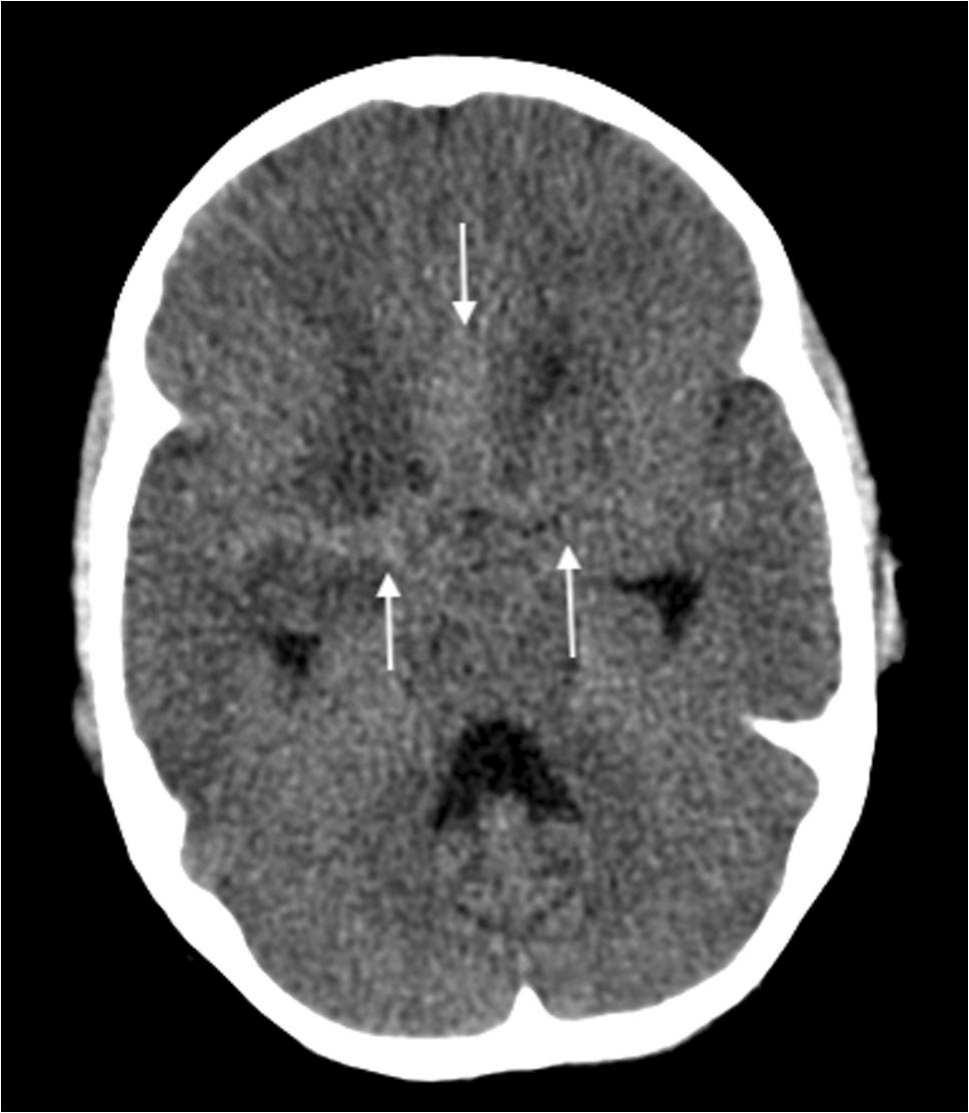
Non-contrast axial computed tomography of the brain in a 30-month-old boy with tuberculosis demonstrates hyperdensity (*arrows*) in the basal cisterns, representing granulation tissue and exudate. Note the bilateral basal ganglia and right temporal lobe low density, indicating established infarcts
